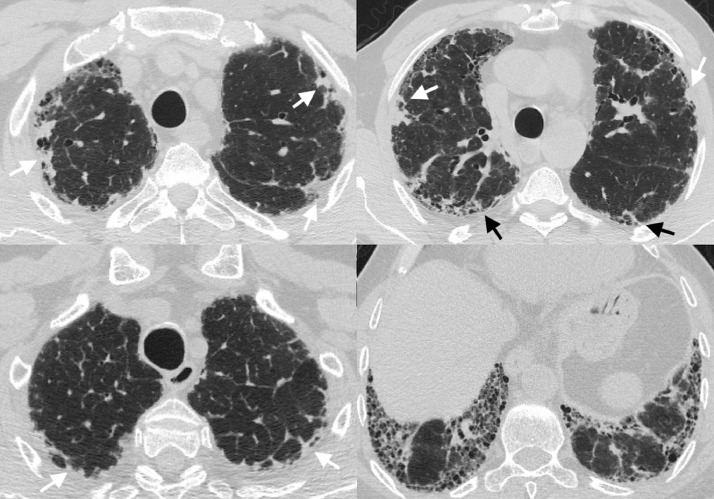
Axial CT images demonstrating upper-lobe predominance of PPFE. Axial CT images demonstrating (top row) a patient with radiological PPFE in upper lobes (white arrows) and apical segments of lower lobes (black arrows) and (bottom row) a patient with radiological PPFE in upper lobes (white arrows) and PPFE absent from lower lobes. PPFE = pleuroparenchymal fibroelastosis.
